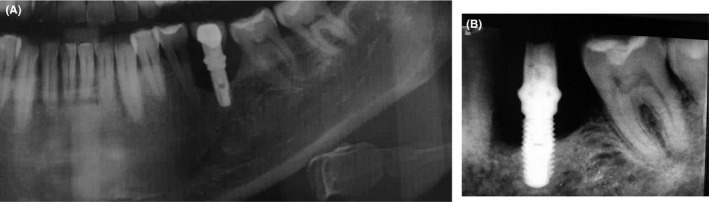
A, panoramic radiograph of implant site B, Intraoral periapical radiograph of implant site
